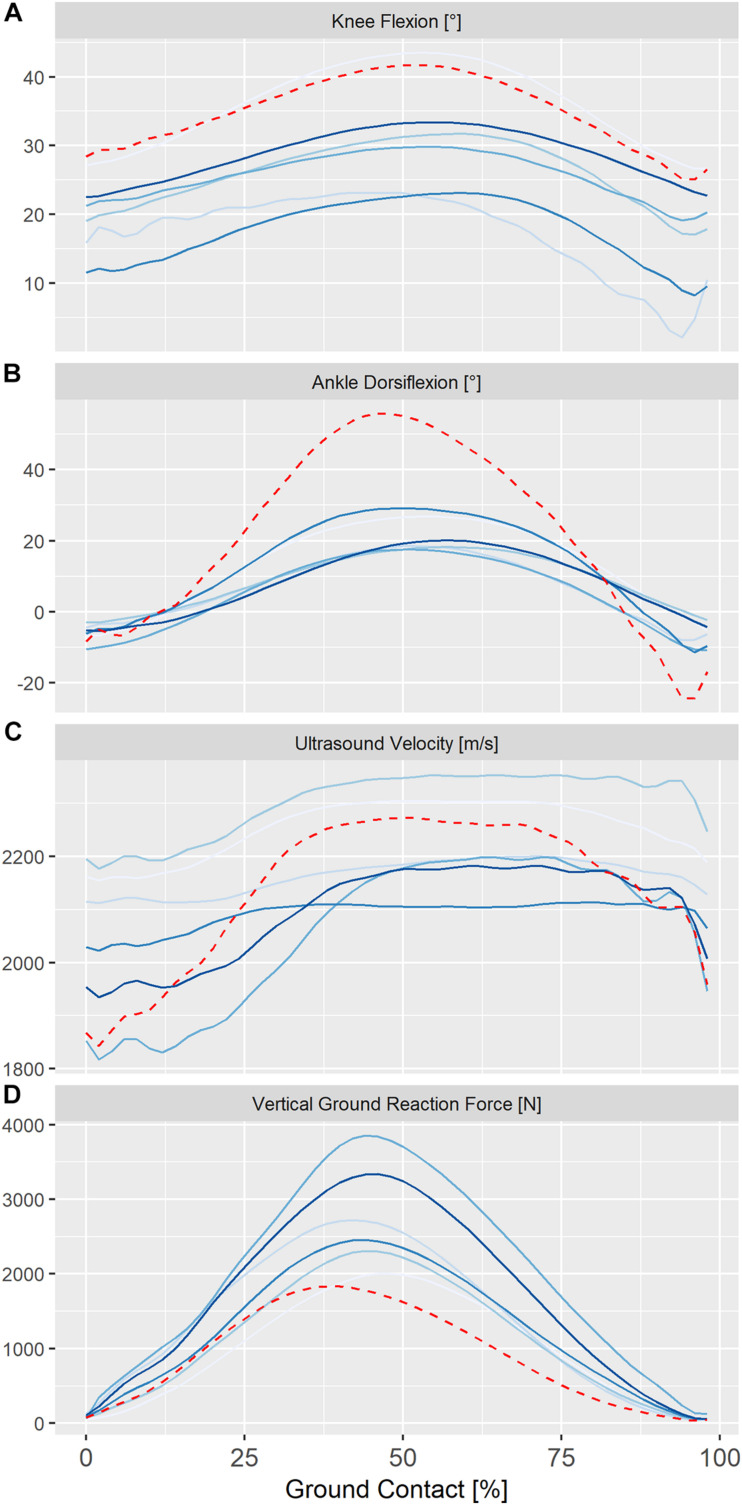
Individual ensemble histories for knee kinematics (A) ankle kinematics (B), ultrasound velocity in the Achilles tendon (C) and vertical ground reaction forces (D) during the ground contact phase of steady-state hopping in six healthy adults (solid lines) and in a single adult, 24 months post-surgical repair (dashed line). Positive values for sagittal knee and ankle kinematics represent flexion and dorsiflexion, respectively.
